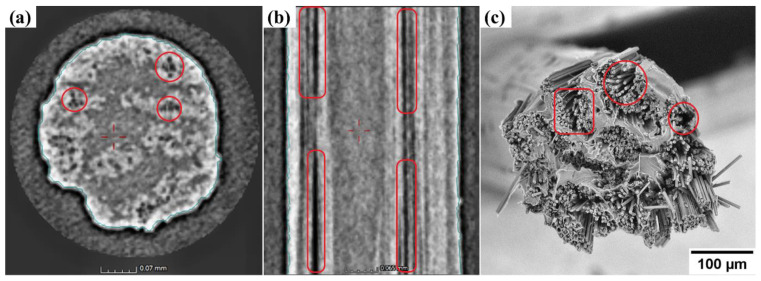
Images acquired via CT scan and SEM. Overview of CCF via CT (a); sectional view of CCF via CT (b); cryofractured surface of CCF via SEM (c).
